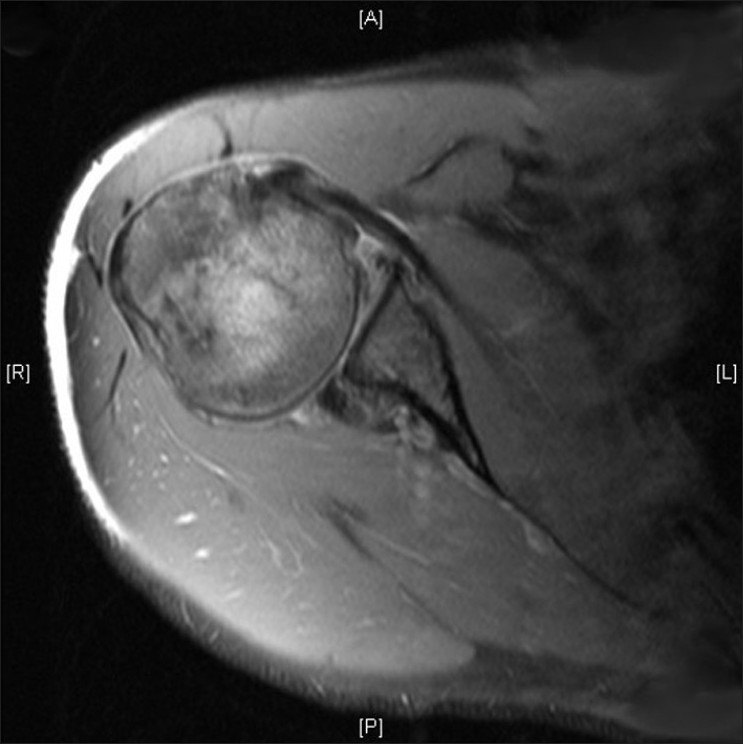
MRI showing glenoid fracture
